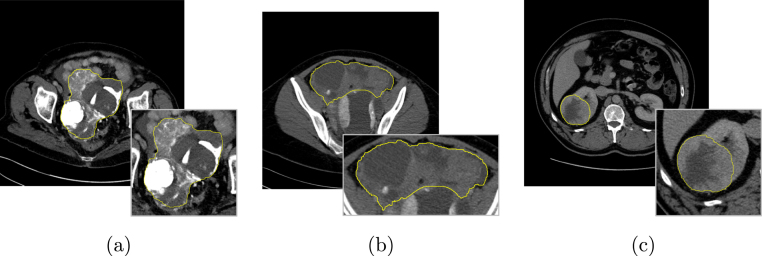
Examples of input axial CT slices for tissue-specific sub-segmentation: (a, b) HGSOC lesions in the pelvis and omentum, respectively; (c) RCC. The whole tumour burden, defined by the yellow contour and zoomed at the bottom right of each sub-figure, is characterised by mixed tumoural tissues.
